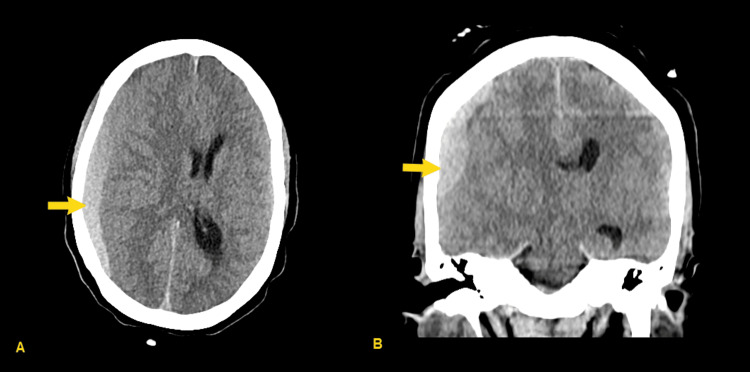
Admission CT head, showing subdural hematoma (SDH) (yellow arrows) with midline shift, subfalcine, and uncal herniation (A) Axial image, (B) Coronal image
